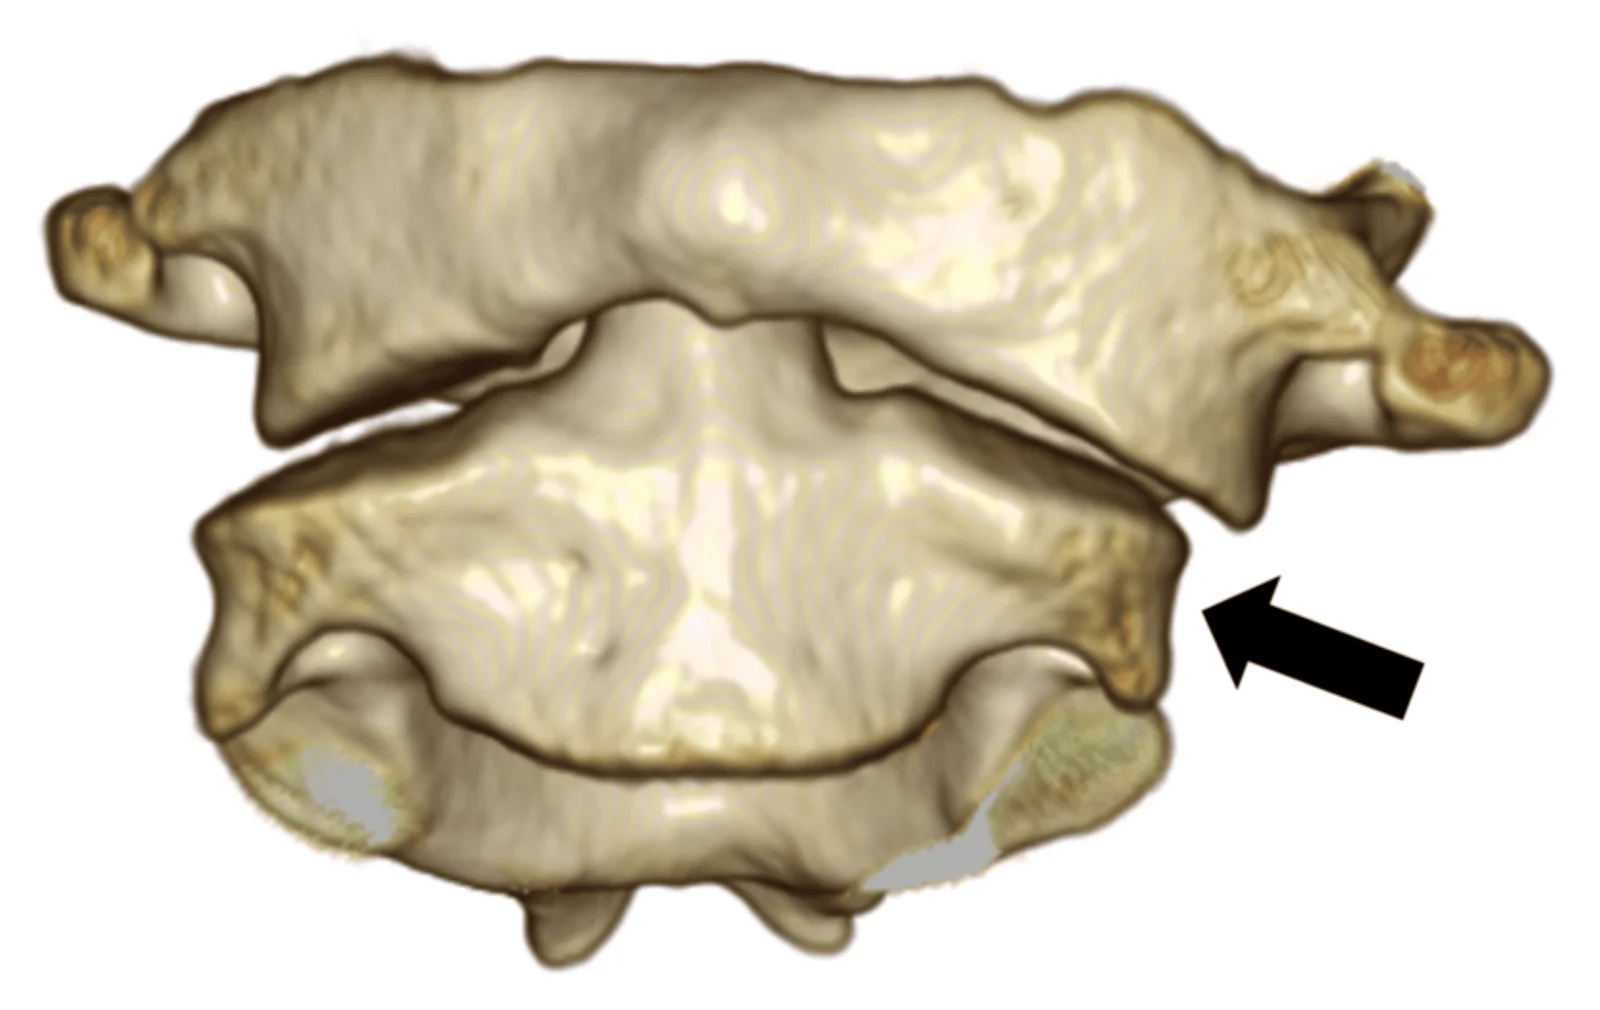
Initial CT findings. Three-dimensional reconstructed CT images obtained at presentation. Despite the two-week interval from symptom onset, mild deformity of the anterior portion of the left C2 facet was already evident. No clival deformity or asymmetry was observed.
